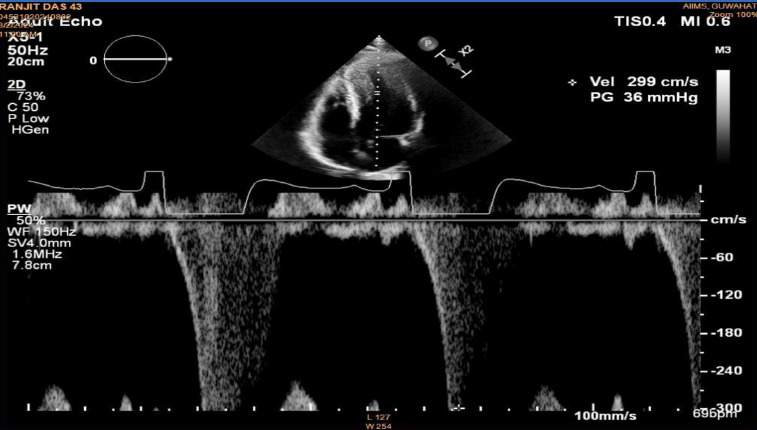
2 D Echocardiography with Pulse Doppler showing mid-cavitary gradient of 36 mm Hg (above 30 mm Hg is diagnostic cut-off for MCO).
